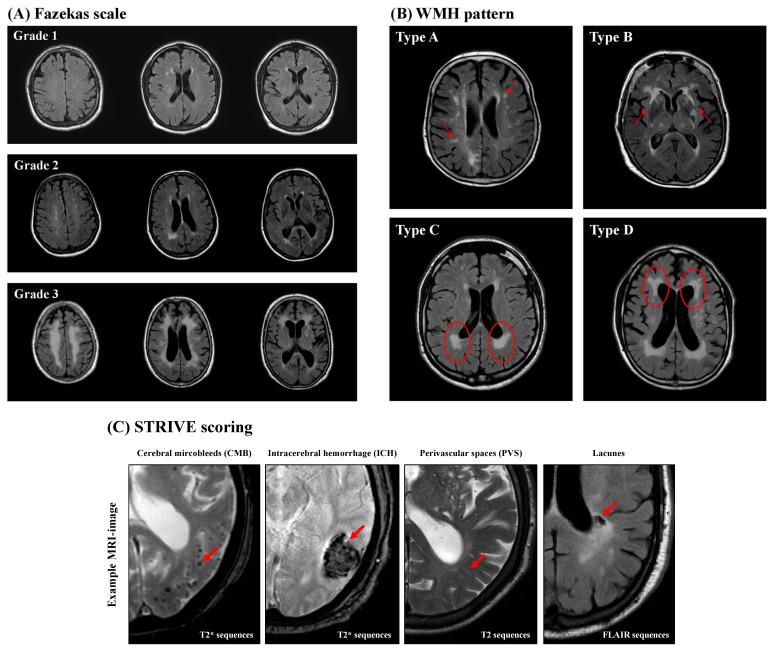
Representative magnetic resonance imaging (MRI). The Fazekas scale (A) is a graduated scoring system in which the degree of white-matter hyperintensity (WMH) increases with the degree of WMH (grades 1–3). The WMH pattern (B) categorizes the distribution of WMH in a quantitative way into four types: multiple subcortical spots (type A), peri-basal ganglia WMH (type B), posterior subcortical pattern (type C), and anterior subcortical pattern (type D). The red circles and red arrows mark the characteristic MRI findings. (C) STRIVE MRI scoring. The red arrows mark the characteristic MRI findings.
